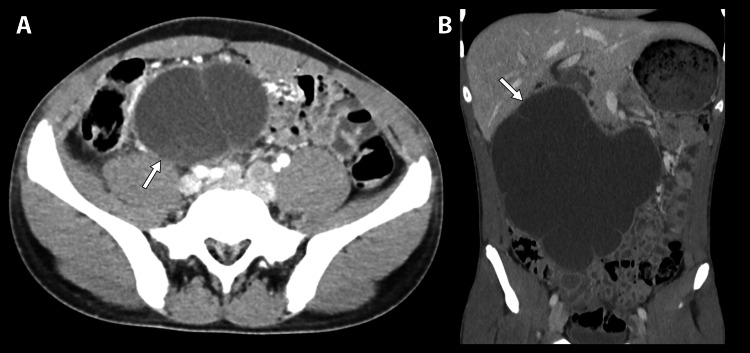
(A) Axial contrast-enhanced CT image showing a thin-walled cystic lesion in the right hemiabdomen. Note the lobulated contour with thin internal septal deviations. (B) Coronal contrast-enhanced CT imaging demonstrating the thin-walled cystic lesion measuring 8 x 10 x 19 cm along the transverse-anteroposterior-craniocaudal axis. The lobulated contour with thin internal septations is visualized.
